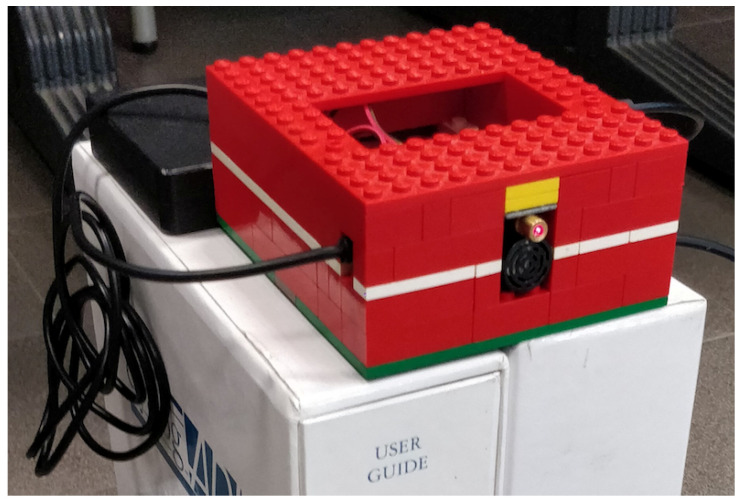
IoT node defined for the experimental characterization campaigns, composed by a Raspberry Pi 3 Model B+, an ultrasonic sensor, and a laser emitter.
